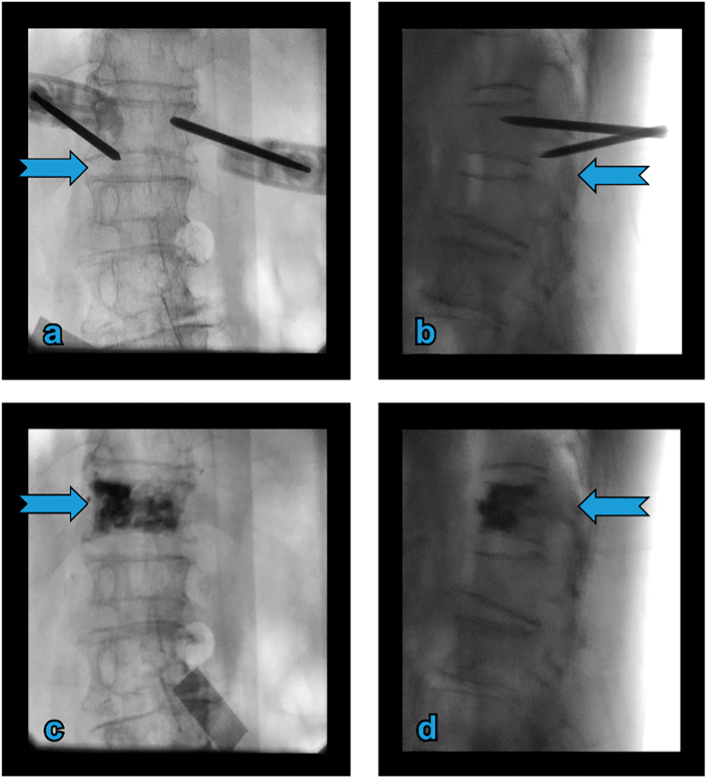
Intraoperative fluoroscopy. (a and b) The left puncture needle accidentally penetrating the intervertebral foramen. (c and d) Anteroposterior view of the lumbar radiograph showing no evident intraspinal cement leakage.
